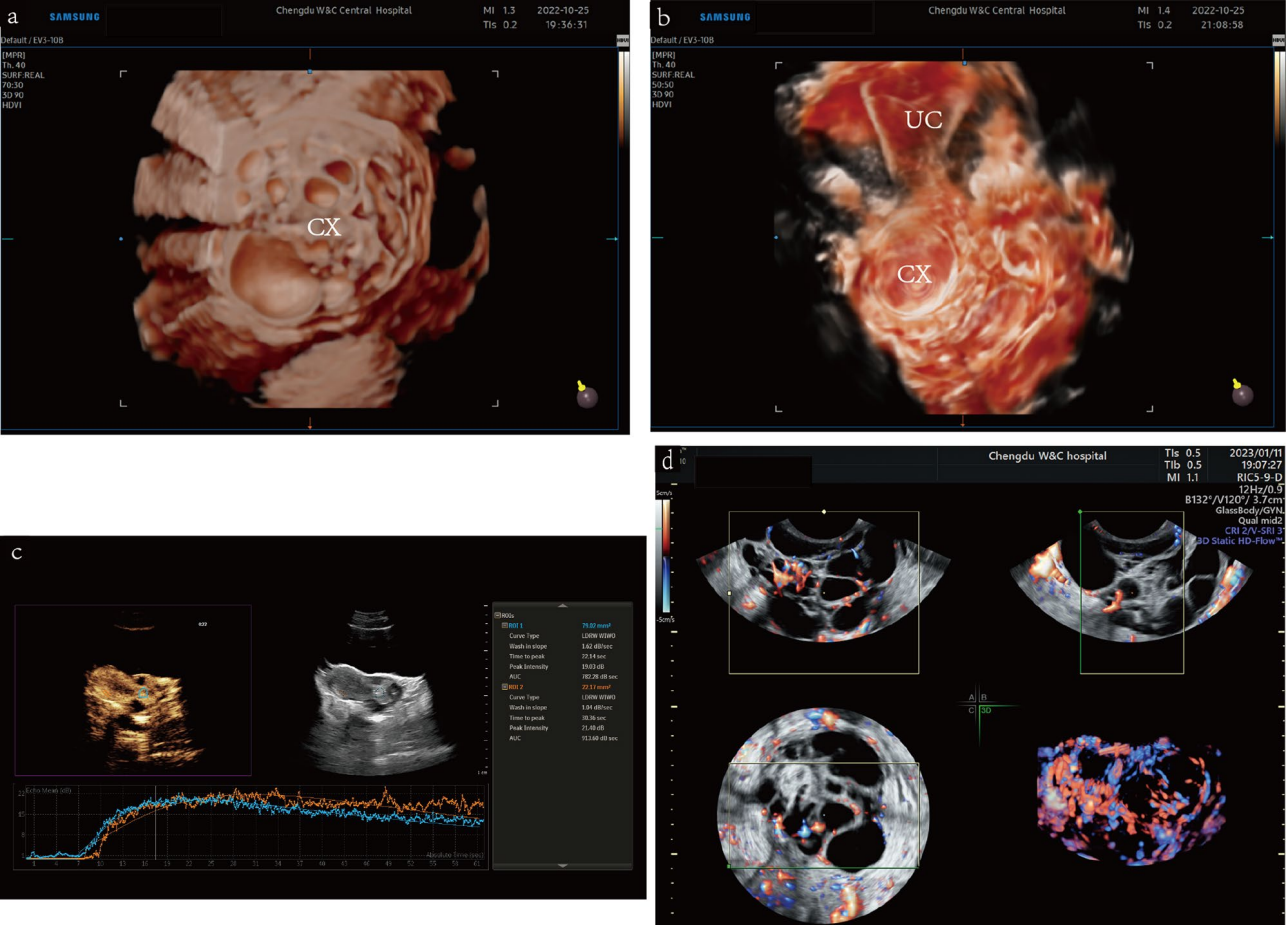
Three-dimensional and contrast-enhanced ultrasonography imaging. (a) Three-dimensional view on 3D realisticVue (Samsung Medical, Seoul, South Korea) shows multilocular signs resembling the “cosmos pattern” in MRI. (b) Three-dimensional reconstructed coronary section of uterus and cervix presented by the CrystalVue software (Samsung Medical, Seoul, South Korea). The enlarged barrel-shaped cervix with multilocular resonances and an uninvaded uterine cavity can be observed. (c) CEUS (PHILIPS EPIQ7, Philips Healthcare, Seattle, WA, the United States) examination on the blood perfusion of the cervical lesion. The ROI and curve in blue indicate the perfused region and TIC of the solid component in the cervix; the ROI and curve in orange represent the perfused region and TIC of the myometrium. The solid components of the cervical mixed echo began developing at the 7th second and peaked at the 22nd second, presenting a pattern of “quick-up and slow-down.” (d) Three-dimensional power Doppler (General Electric Company, Boston, Massachusetts, the United States) displays the increased blood supplies with irregular ramification, part of which multiplies and forms a clump-like pattern. *Abbreviation* CEUS, contrast-enhanced ultrasonography; CX, cervix; EN, endometrium; MRI, magnetic resonance imaging; ROI, region of interest; TIC: time-intensity curve
